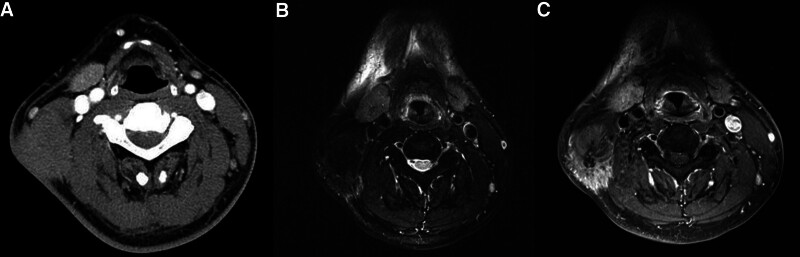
(A) CT, axial homogeneous enhancing mass infiltrating the sternocleidomastoid muscle and skin (B) MRI T2WI shows a mass infiltrating the SCM muscle with low signal intensity (C) MRI TIWI images shows a mass with similar signal intensity to muscle and had heterogeneous enhancement. CT = computed tomography, MRI = magnetic resonance imaging, SCM = sternocleidomastoid.
